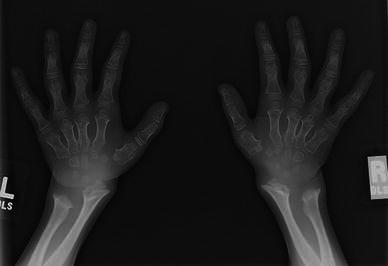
Hand and wrist changes in Morquio A syndrome. Carpal ossification delay, short and broad metacarpals, metacarpal rounding, and a short ulna are evident in the radiograph of an 8-1/2-year old female.
